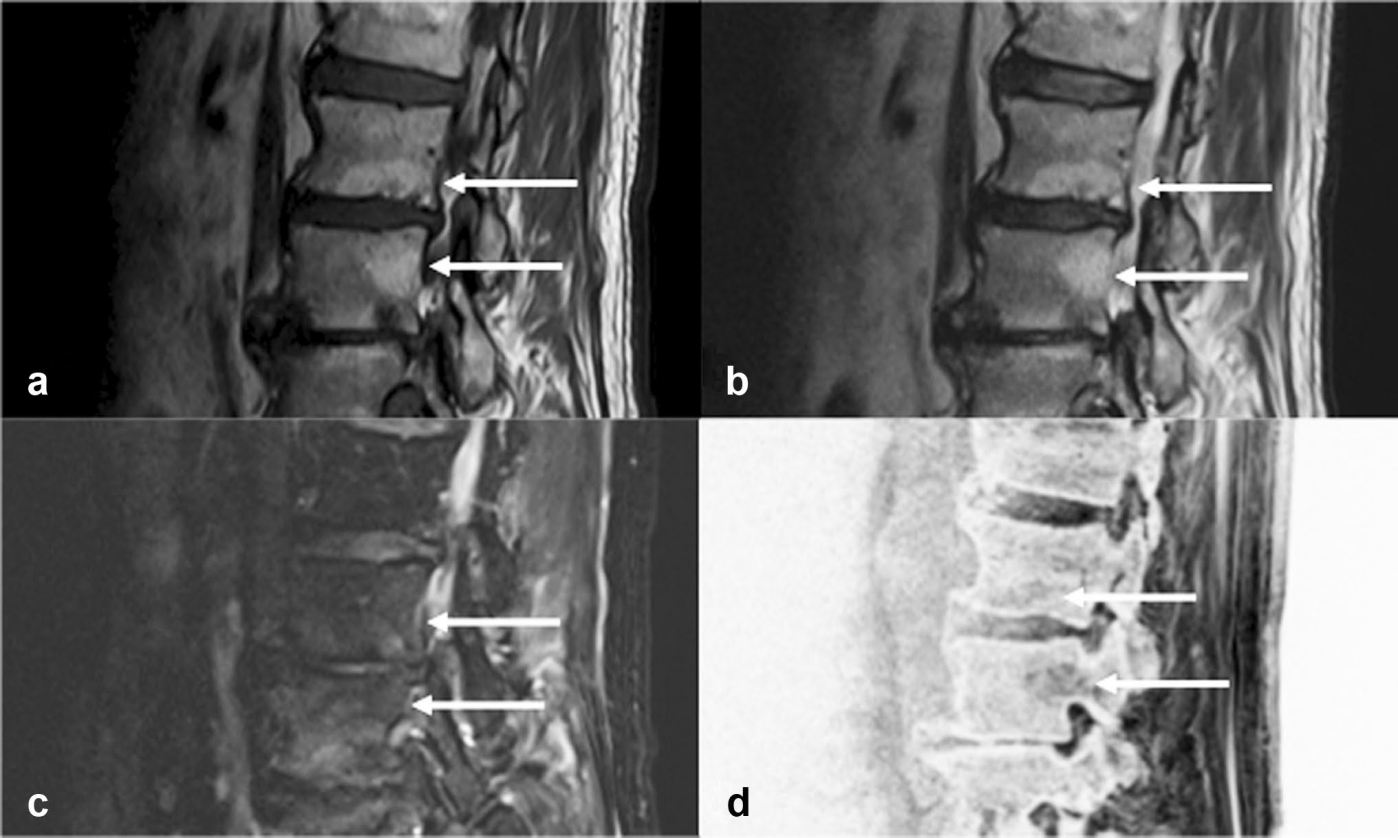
Modic type 2 change. Modic type endplate changes represent a classification for vertebral body endplate signal changes on MR imaging. Modic type 2 change represents red hemopoietic bone marrow conversion to yellow fatty marrow due to bone marrow ischemia. Sagittal T1-weighted (a), T2-weighted (b), and fat-suppressed T2-weighted (c) MR images of an 84-year-old male patient show signal changes compatible with fatty changes in the bone marrow (arrows). Sagittal MR bone image d shows sparse trabeculae (arrows)
